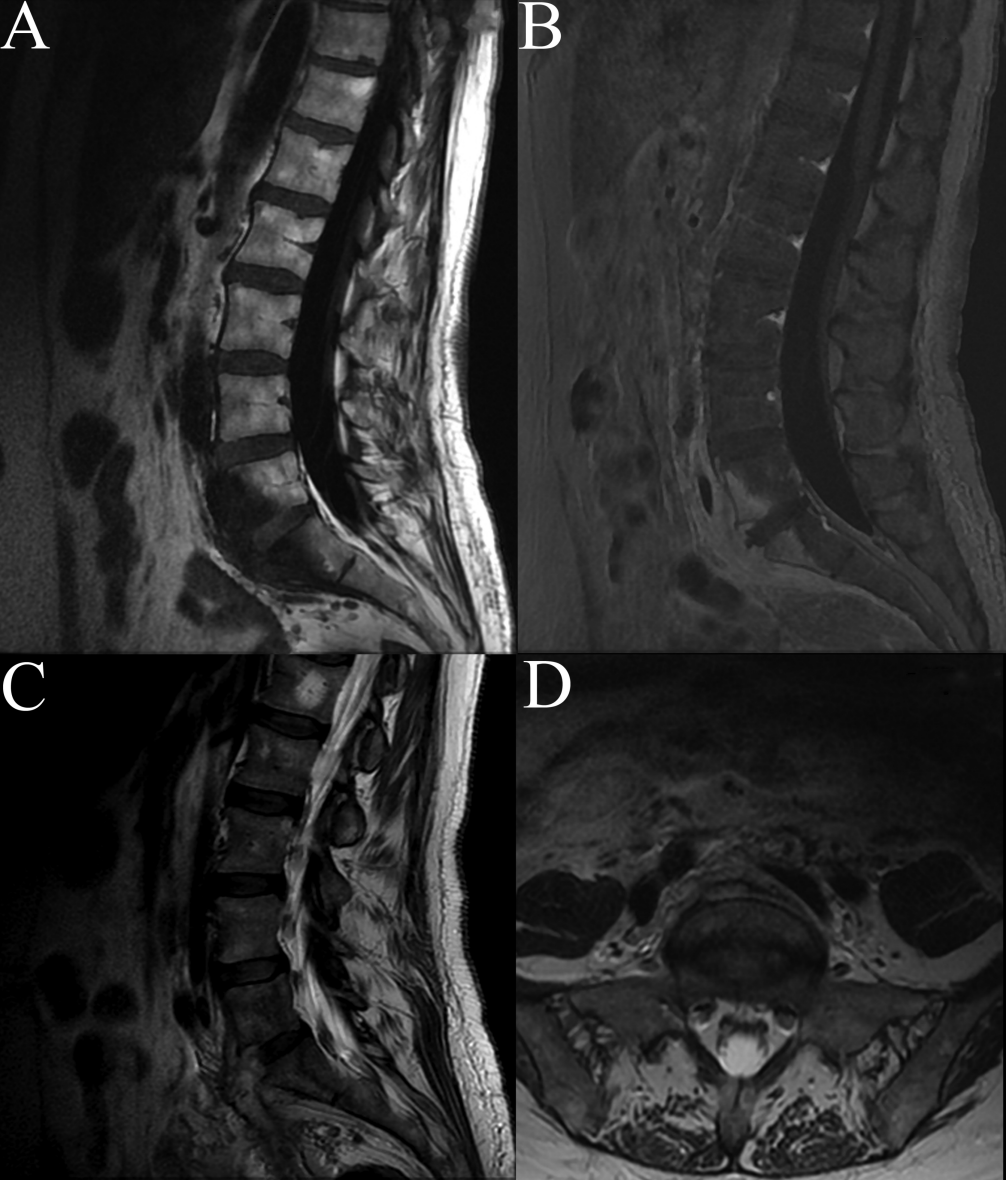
MRI Lumbar Spine Figure 1: MRI of lumbar spine with sagittal T1 (A) and contrasted T1 (B) with enhancement in the L5 and S1 bodies posterior to a hyperintense soft-tissue mass seen on T2 sagittal (C) and axial (D).
